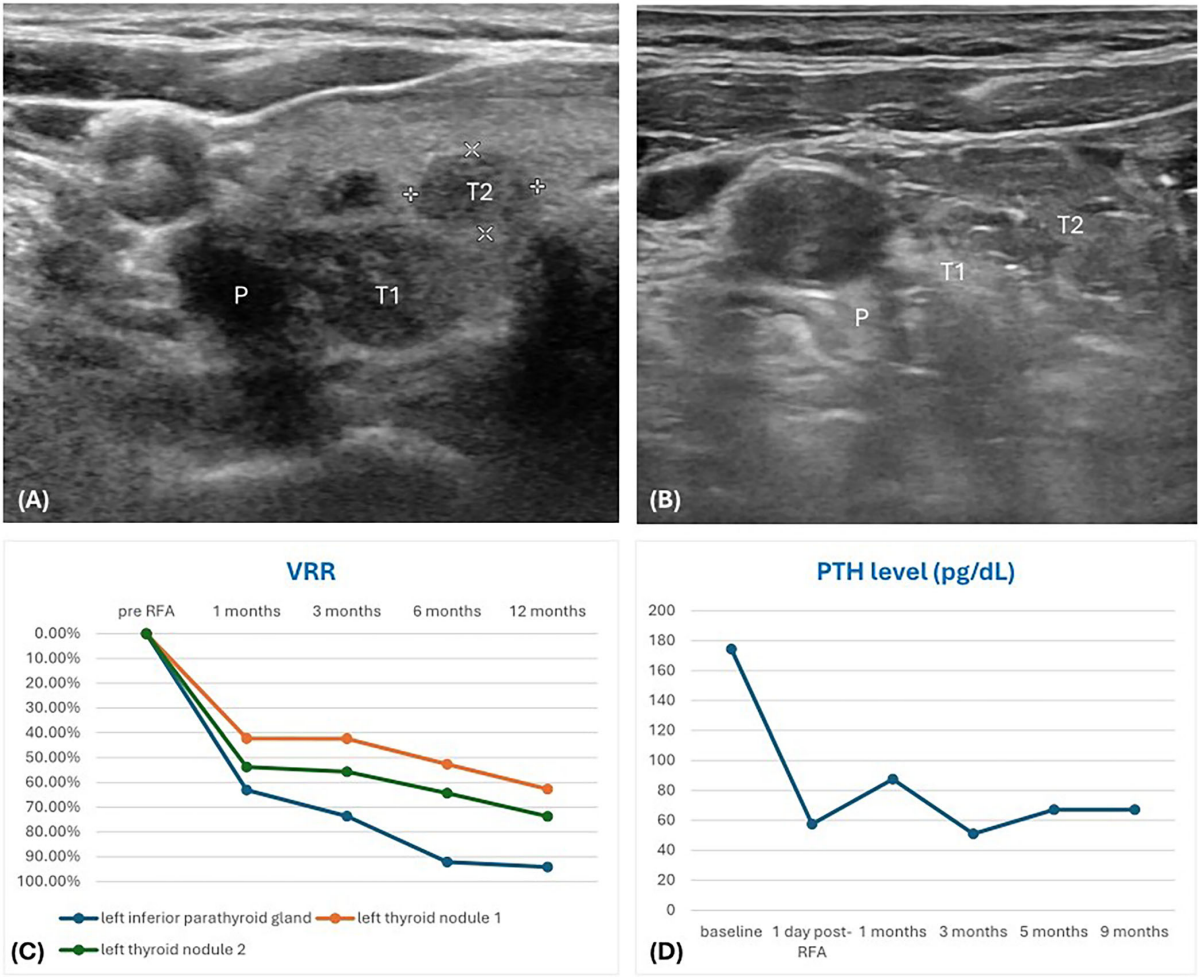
(A) Pre‐RFA ultrasound examination. Thyroid nodules (T1 and T2) and parathyroid adenoma (P) were marked. (B) Post‐RFA ultrasound showed hyperechoic feature. (C) VRR during the follow‐up period. The post‐RFA VRR of parathyroid adenoma at 12 months was 94.1%, and that of T1/T2 thyroid nodules was 62.7%/73.7%, respectively. (D) PTH level. The baseline level was 174.5 pg/mL. The post‐RFA PTH level at 9 months was 67.2 pg/mL. PTH, parathyroid hormone; RFA, radiofrequency ablation; VRR, volume reduction ratio.
